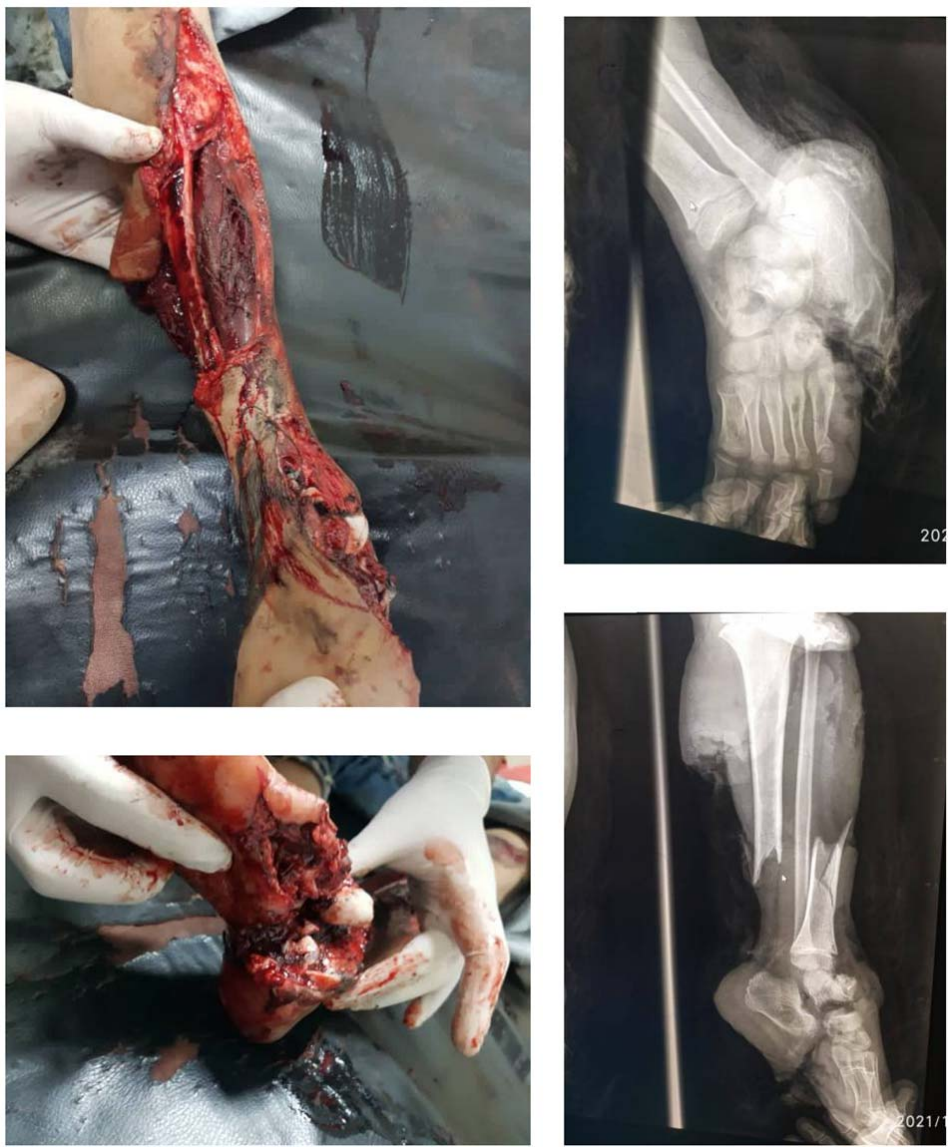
Displays the fractured image alongside the X-ray images taken prior to the intervention.
